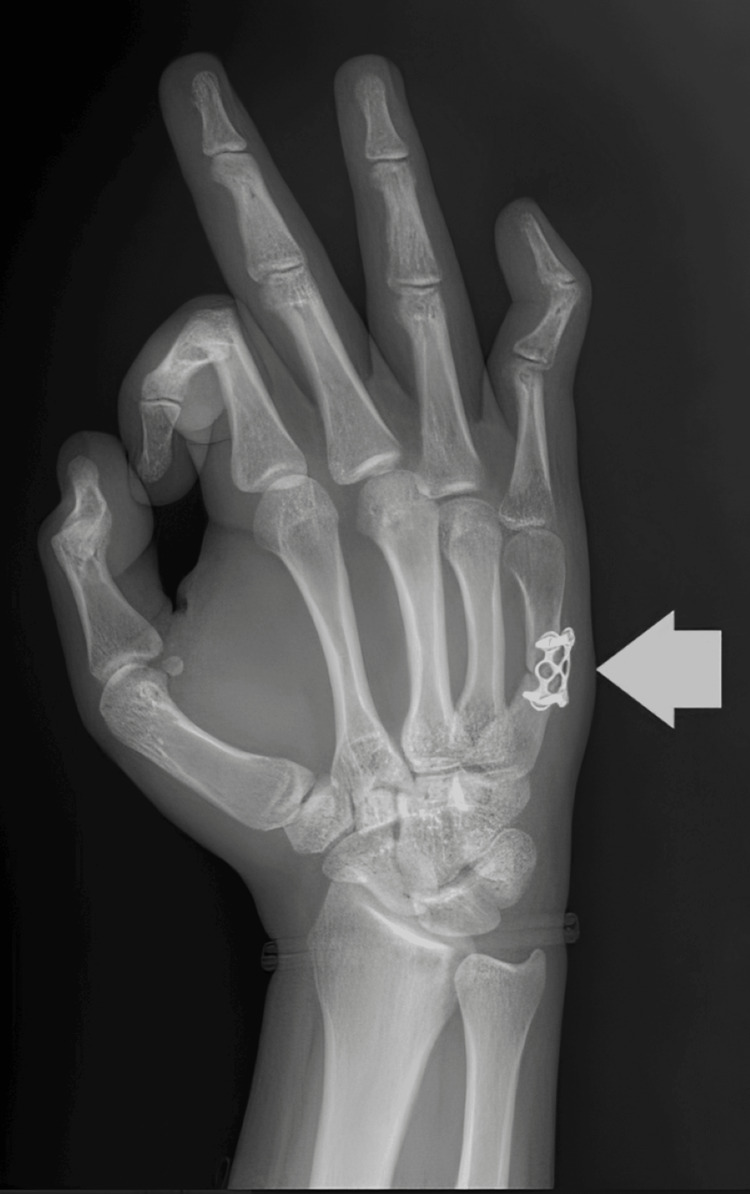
Radiograph image of the right hand. The white arrow indicates a titanium miniplate fixed to the shaft of the fifth metacarpal.
